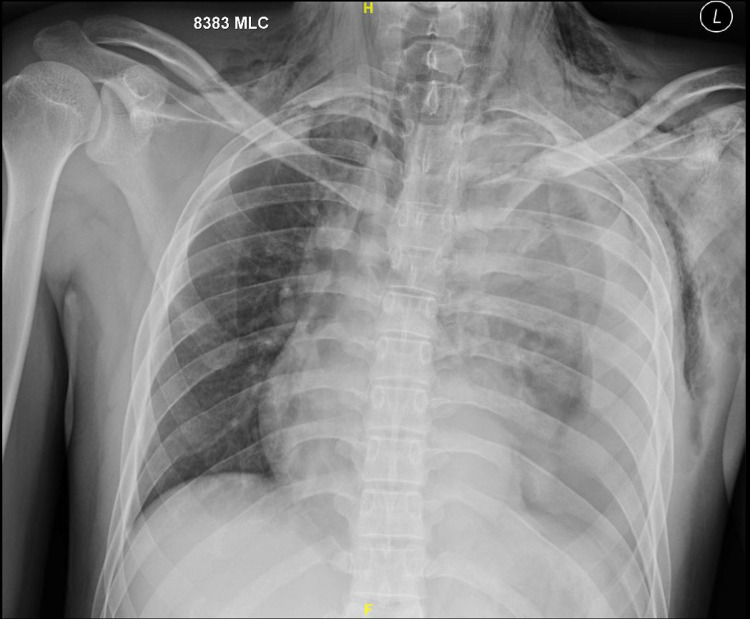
Chest X-ray showing left-sided hemo-thorax with left clavicle, left first, second, and third rib fracture.
